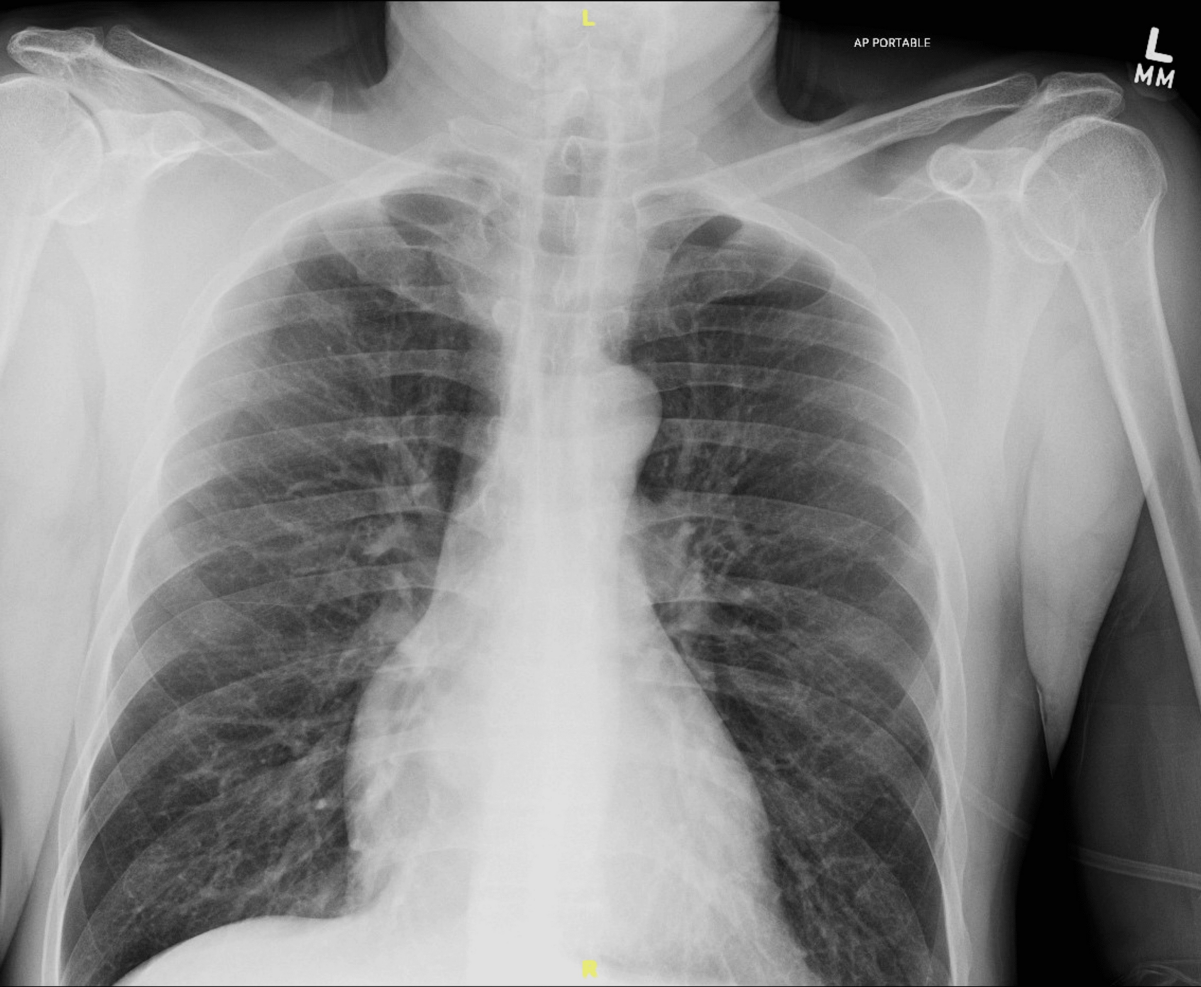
A portable supine chest anteroposterior X-ray showing pulmonary hyperinflation with air trapping.
